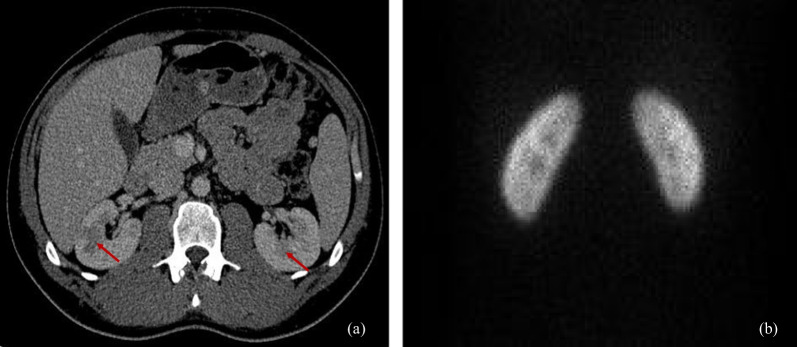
Normal-sized kidneys with multiple bilateral hypodense changes, largely involving the kidney medulla (left and right red arrows are pointing to hypodense changes suspected of renal infarction that measure 16 mm and 25 mm in diameter, respectively. (a) Static (cortical) renal scintigraphy showed complete regression of renal lesions visualized on abdominal CT (b)
